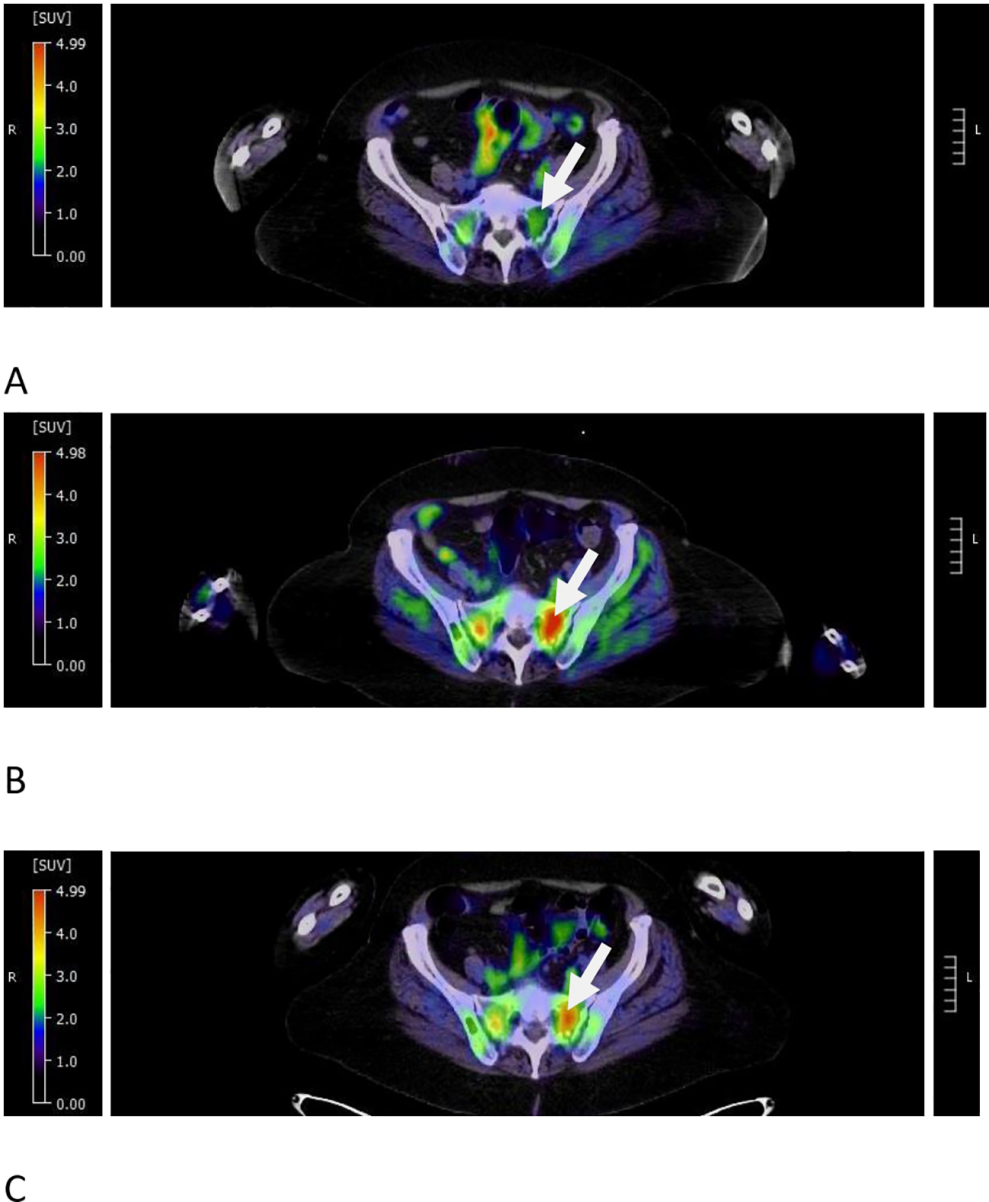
Pseudoprogression in target FDG avid pelvic lesion during teclistamab treatment. (A) At 9 months from the start of therapy, the left sacral lesion had a decreased SUVmax of 3.24 from 9.5 at relapse of the disease (see the arrow). (B) At 12 months from the start of therapy, the same dominant lesion demonstrated increased SUVmax to 5.11 (see the arrow). (C) At 3 months after the discontinuation of therapy, the SUVmax showed a minimal interval decrease to 4.7 (see the arrow).
